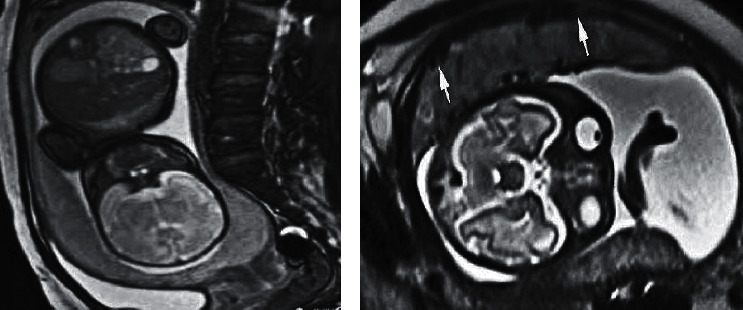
The MRI image of a complete placenta previa case. (a) The sagittal plane. (b) The transverse plane.
